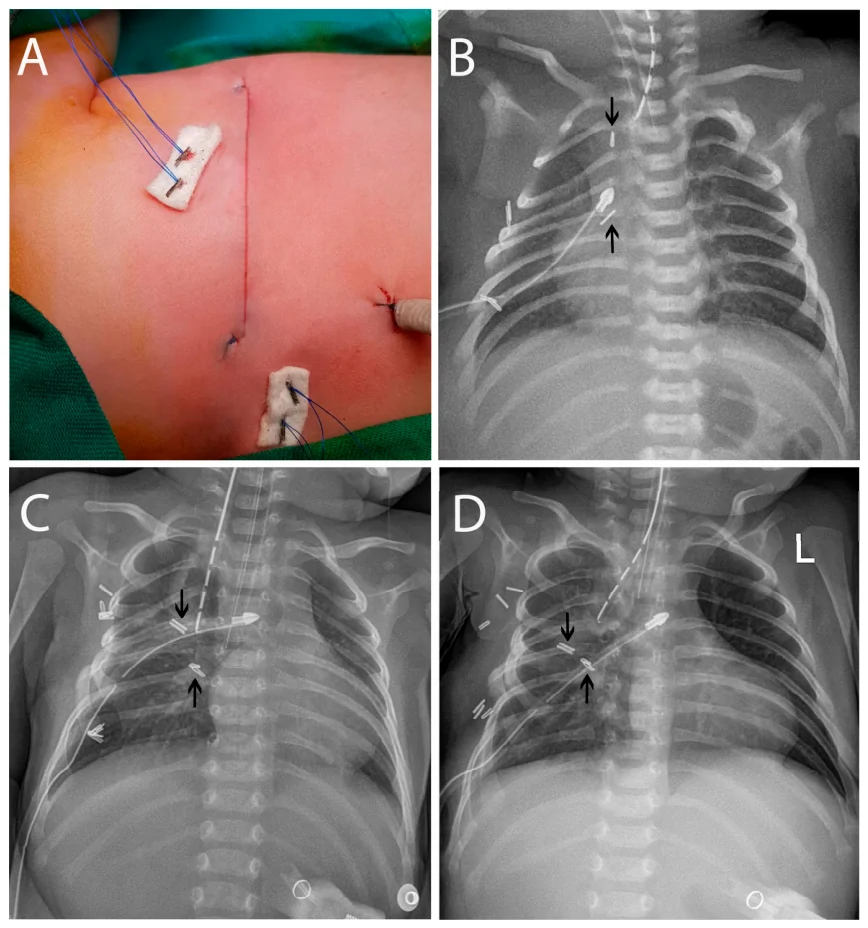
External traction and stepwise reduction in the esophageal gap during the Foker procedure. Traction sutures are externalized through the chest wall, secured to the skin, and periodically retightened (A). Serial chest radiographs demonstrate progressive reduction in the esophageal gap (B–D); arrowheads indicate the metallic clips marking the ends of the proximal and distal esophageal pouches. L, left.
